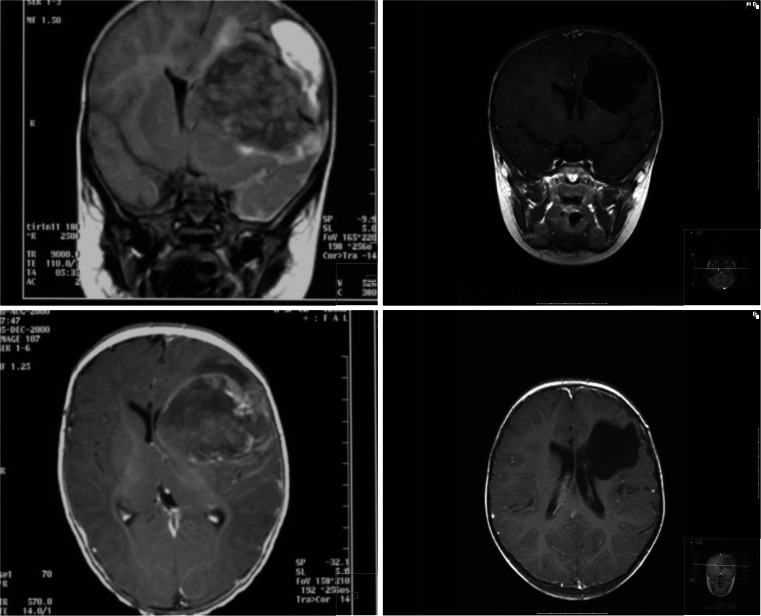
Patient 15. Four-month-old girl with acute symptoms (seizures) due to a hemispheric PNET with acute bleeding. She underwent GTR followed by chemotherapy. She has a 14-year event-free survival. Coronal and axial preoperative and postoperative MRI scans are shown
